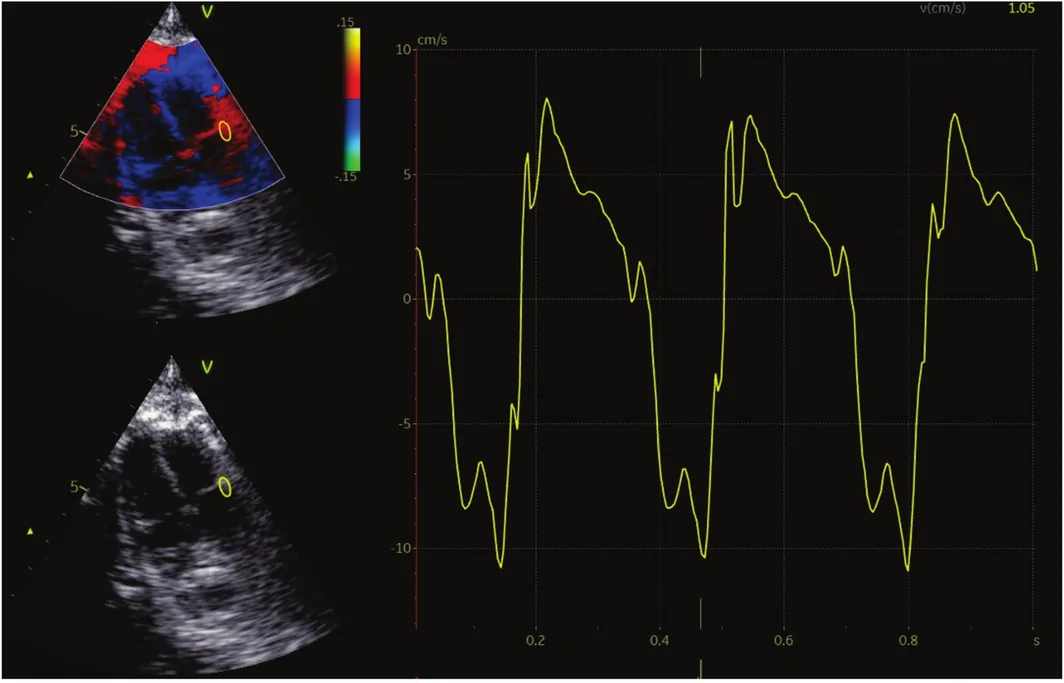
Placement of the region of interest (6 × 3 mm) on the fetal right ventricular wall at the atrioventricular valve plane level to measure the color tissue Doppler imaging (cTDI) waveform with EchoPAC. This waveform was then exported and transferred to MATLAB for analysis by automated algorithm (Figure 3).
